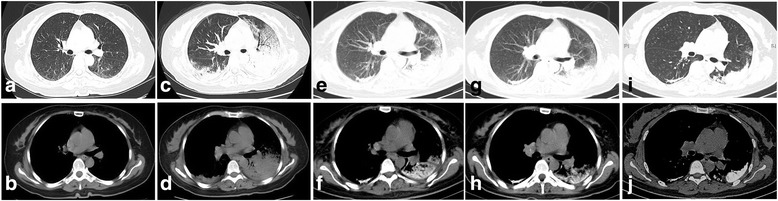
CT scanning of the chest. a, b Initial CT scans: A little linear opacity on the bilateral pulmonary. c, d CT scans five days later: Bilateral pulmonary multiple high-density shadow with mass consolidation and exudation; obvious progression when compared with initial scan. e, f Chest image after treatment of two weeks: Obvious calcification in bilateral lungs. g, h Chest CT after left parathyroidectomy: Lesions were mildly improved. i, j Pulmonary calcifications did not deteriorate or improve 8 months later in the follow-up examination
